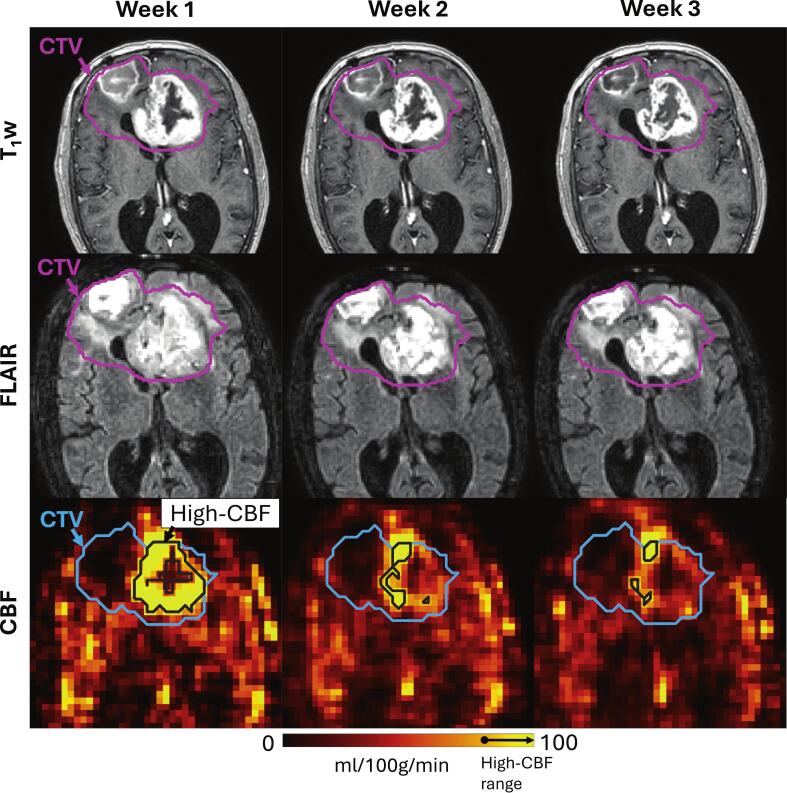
An example of high-CBF region dynamics. T_1_-weighted imaging, T_2_-FLAIR imaging, and CBF maps over three weeks of radiotherapy for a 75-year-old female glioblastoma patient treated with 52.5 Gy/15. The planning CTV is shown in magenta and blue in the anatomical imaging and CBF maps, respectively. The high-CBF region is the black contour on the CBF maps; the arrow on the color bar shows the range of values included in the high-CBF region (>79 ml/100 g/min). The CBF maps reveal substantial decreases in the volume of the high-CBF tumour over time (week 1, 41 cm^3^; week 2, 26 cm^3^; week 3, 11 cm^3^) with minimal change visible in the anatomical imaging. (For interpretation of the references to color in this figure legend, the reader is referred to the web version of this article.)
